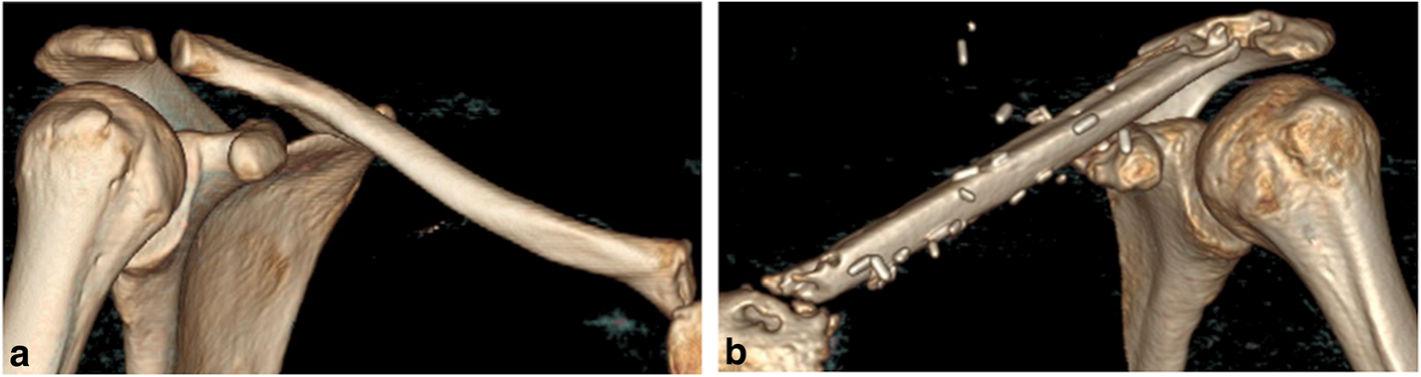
Volume-rendered computed tomography images of a right and b left clavicles after surgical reconstruction of the left clavicle
